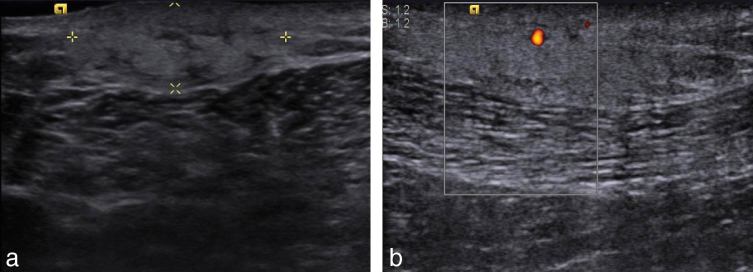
B-mode ultrasound (a) and Doppler (b) images of the left breast reveal a hyperechoic subcutaneous mass with internal vascularity.
